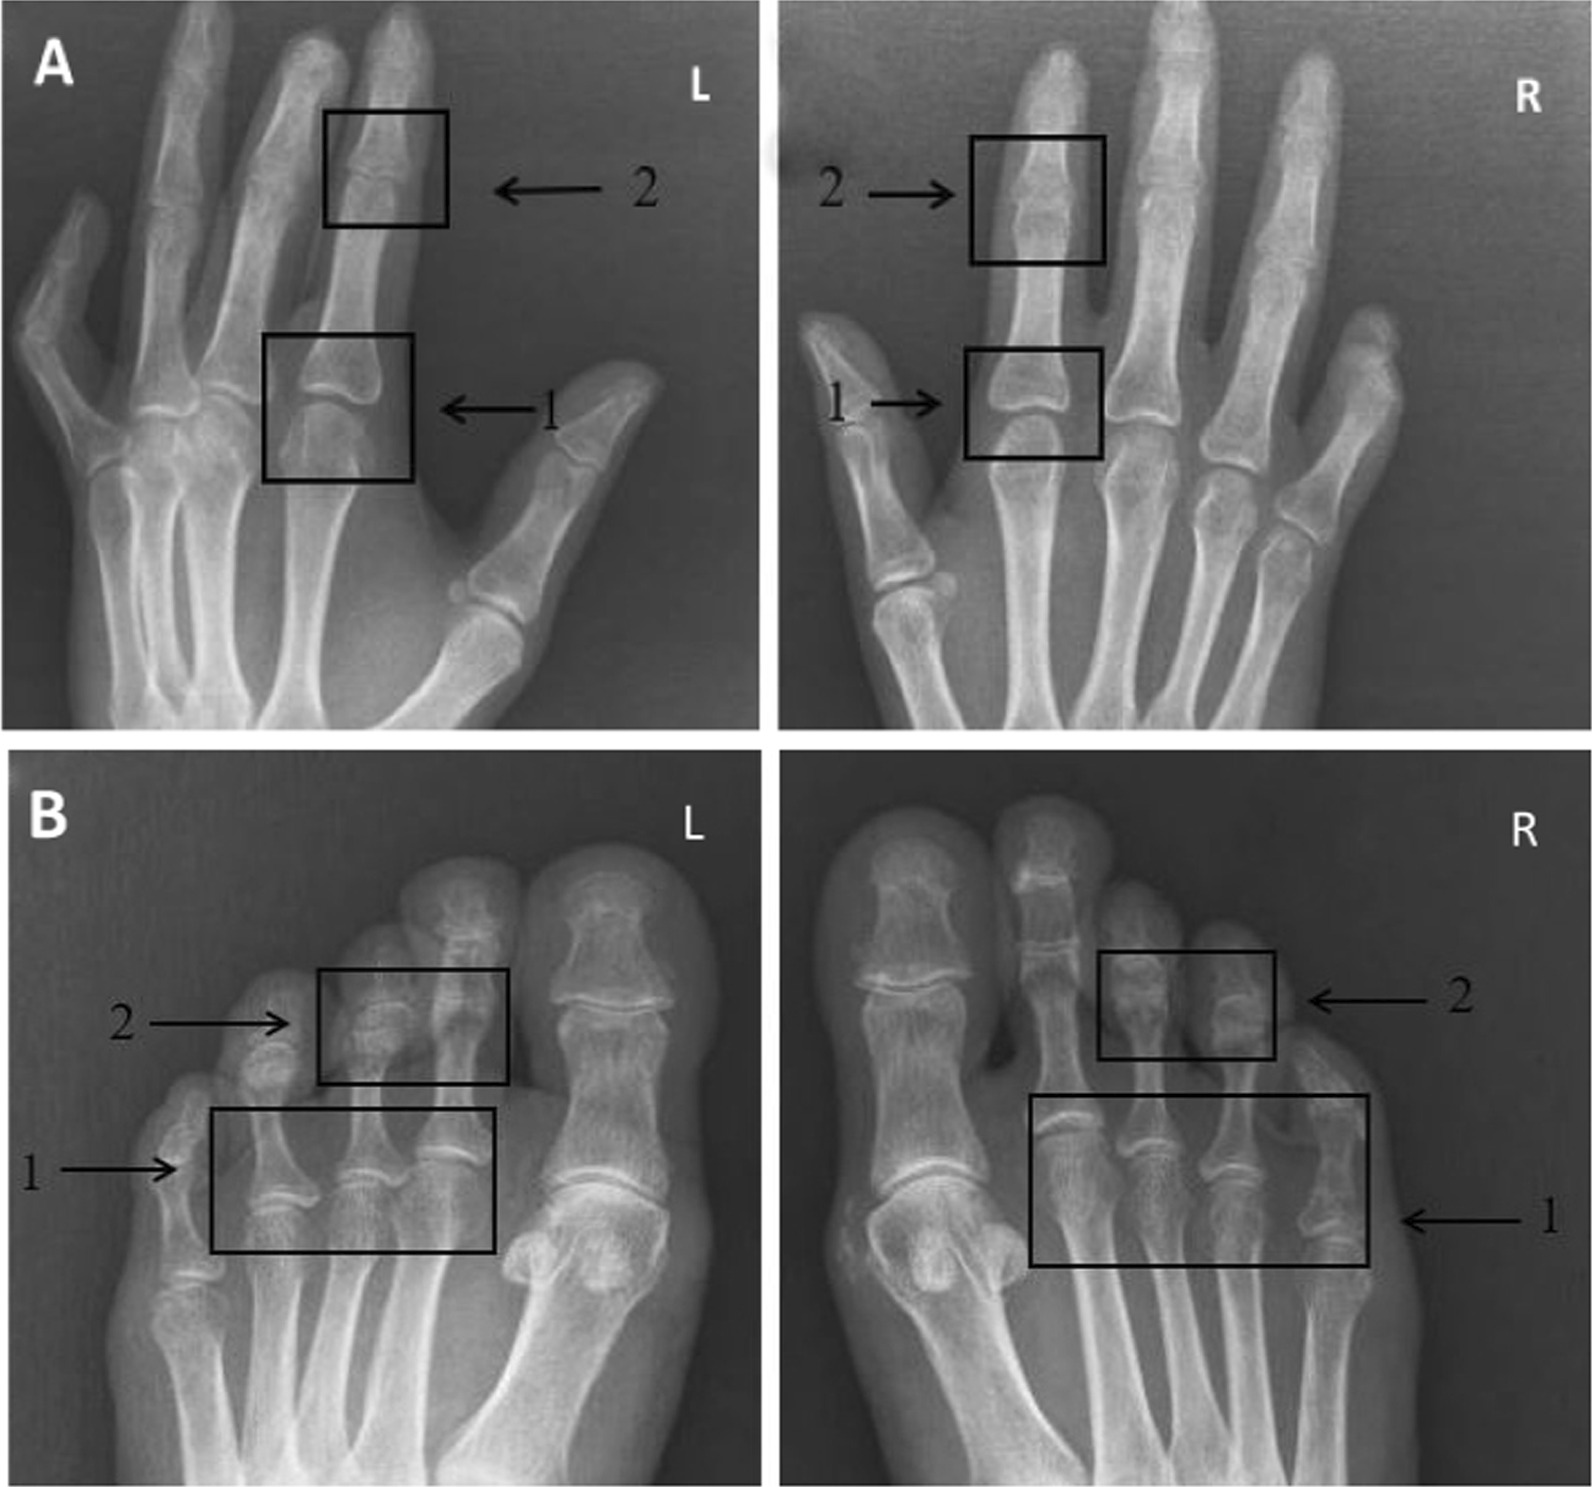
A Hands radiographic images. B Foot radiographic images. A 46-year-old woman with SSc presented with joint pain. Hands and foot radiographic images show the following abnormality (arrows) on the left and right: 1-Bone erosion representing Juxta-articular Osteoporosis 2-Joint Space Narrowing with an abnormal distance between bones
